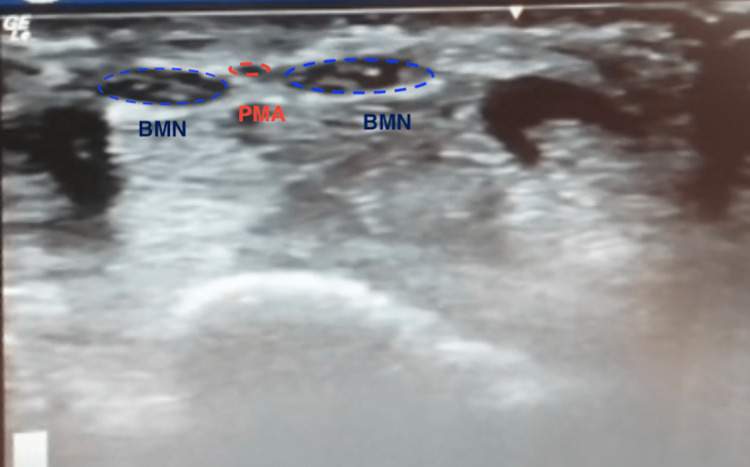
Sonographic imaging of the bifid median nerve and persistent median artery bifid median nerve (BMN), persistent median artery (PMA)
